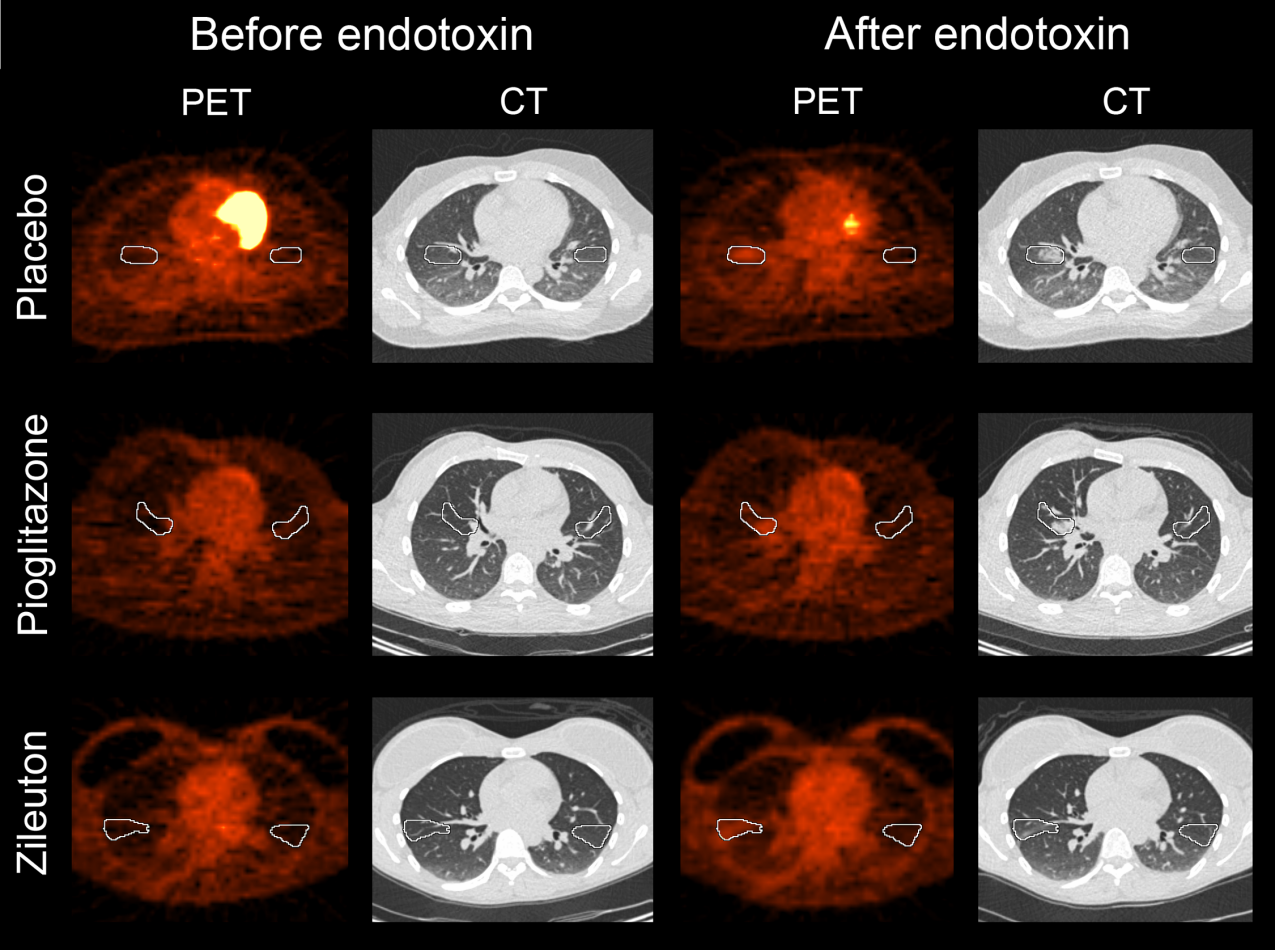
Positron emission tomography (PET) and computed tomography (CT) images from a representative volunteer in each treatment cohort. White outlines show the volumes of interest (VOIs) used to determine the time-activity curves for the Patlak graphical analysis and standard uptake values. VOIs were sometimes smaller in volume on the left due to the heart.
